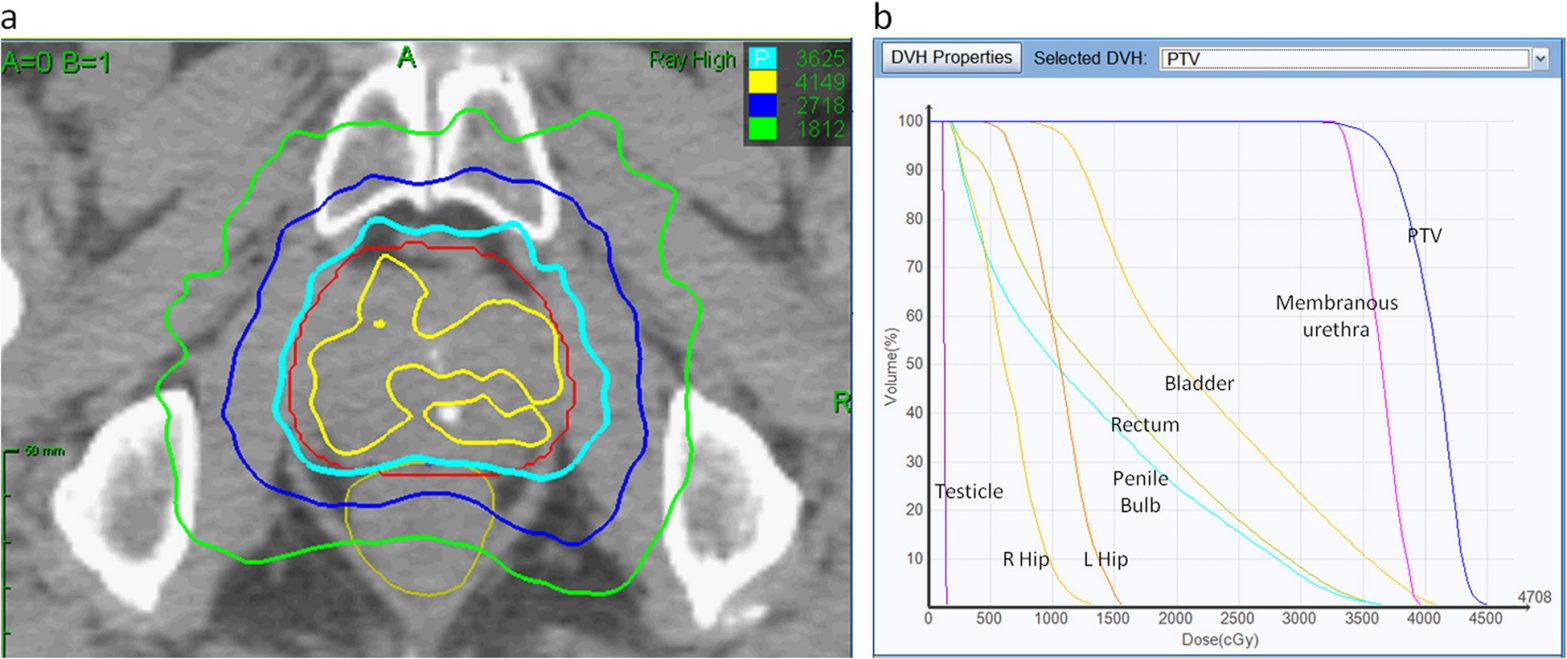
(a) Treatment planning axial computed tomography images demonstrating the prostate (red line) and rectum (brown line). Isodose lines shown as follows: 115% of the prescription dose, yellow line; 100% of the prescription dose, light blue line: 75% of the prescription dose, dark blue line; and 50% of the prescription dose, green line. (b) A typical dose–volume histogram for CyberKnife treatment of a prostate cancer patient.
